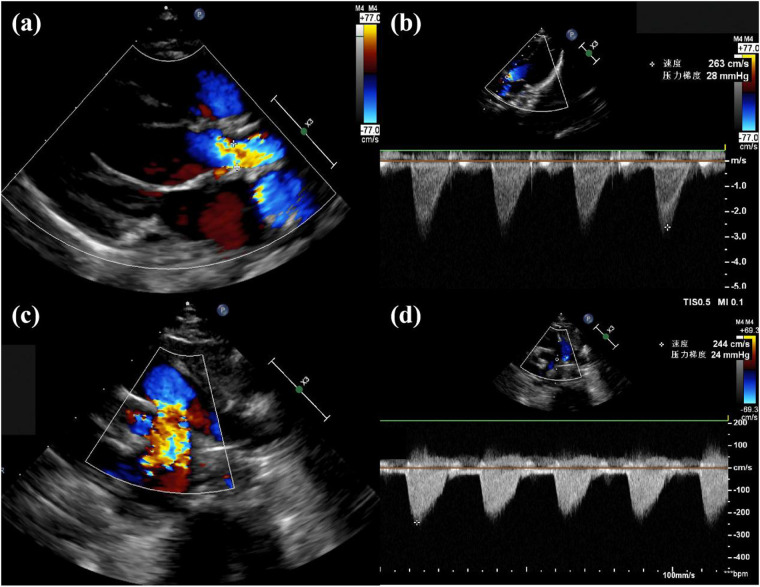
TTE findings of the infant at 7 months of age. (a) Color Doppler imaging showing aliased flow at the narrowed sinotubular junction; (b) Pulsed-wave Doppler demonstrating increased flow velocity at the sinotubular junction; (c) Color Doppler imaging revealing aliased flow at the focal stenosis of the right pulmonary artery ostium; (d) Pulsed-wave Doppler indicating elevated flow velocity in the right pulmonary artery.
